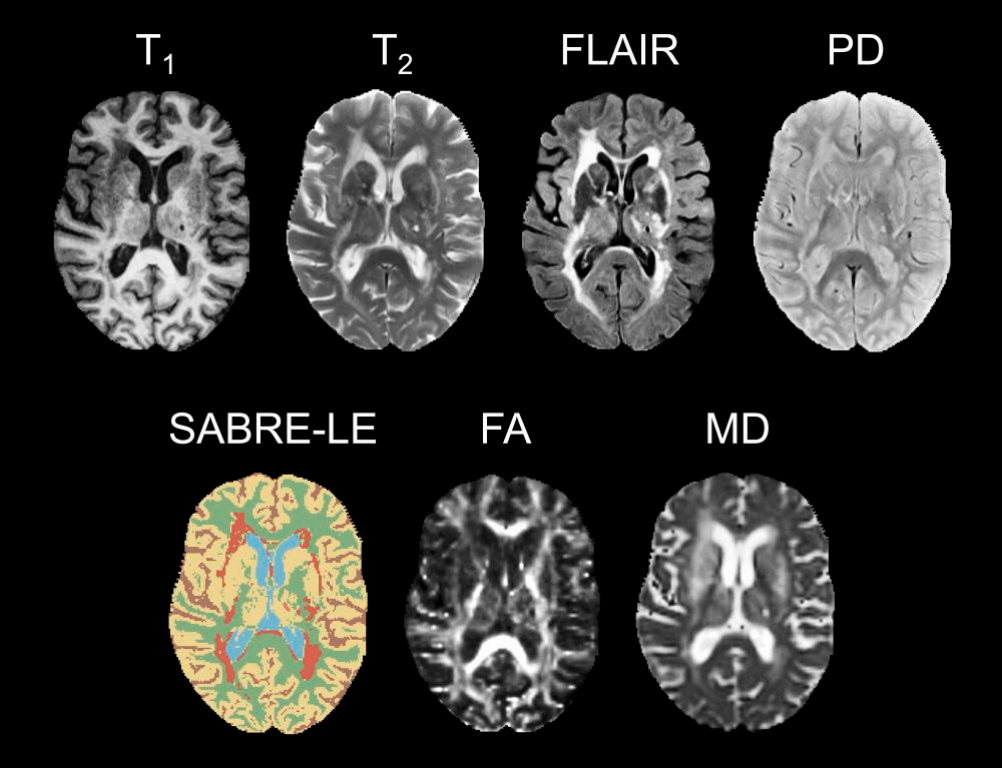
Sample MR images. Examples of the various types of MR images acquired (T_1_, T_2_, FLAIR, PD) or generated (SABRE-LE, FA, MD) in the current study are shown for a single participant with cerebrovascular disease. The SABRE-LE image shows the segmentation of normal-appearing grey matter (beige), normal-appearing white matter (green), white matter hyperintensities (orange), periventricular lacune (lime green), ventricular CSF (blue), and sulcal CSF (brown). FLAIR = fluid-attenuated inversion recovery; PD = proton density; SABRE-LE = Semi-Automatic Brain Region Extraction-Lesion Explorer^52^; FA = fractional anisotropy; MD = mean diffusivity.
